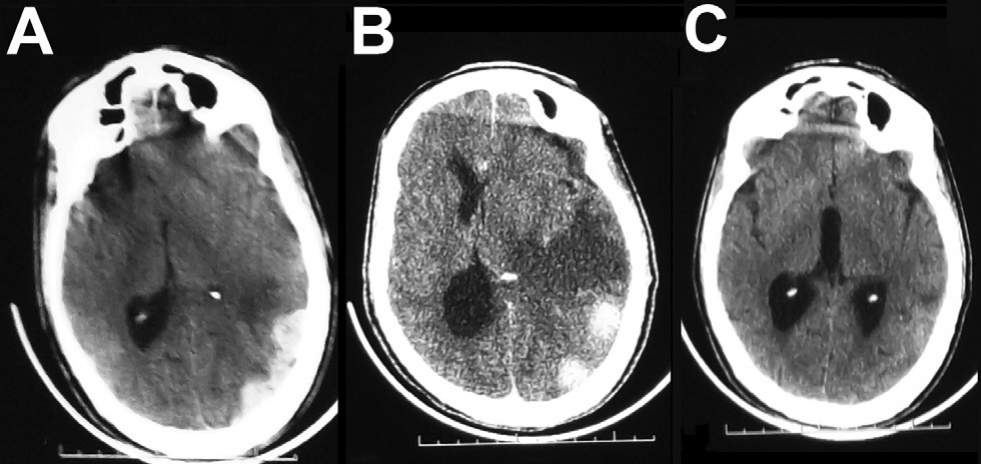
Computed tomography scan image of the brain of a 58-year-old man with Crimean-Congo hemorrhagic fever, Mauritania, 2012. A) Acute subdural hematoma, on the left side. B) Subdural hematoma with perihematomal edema and midline shift. C) Complete resorption of the subdural hematoma with residual edema, 1 month later.
